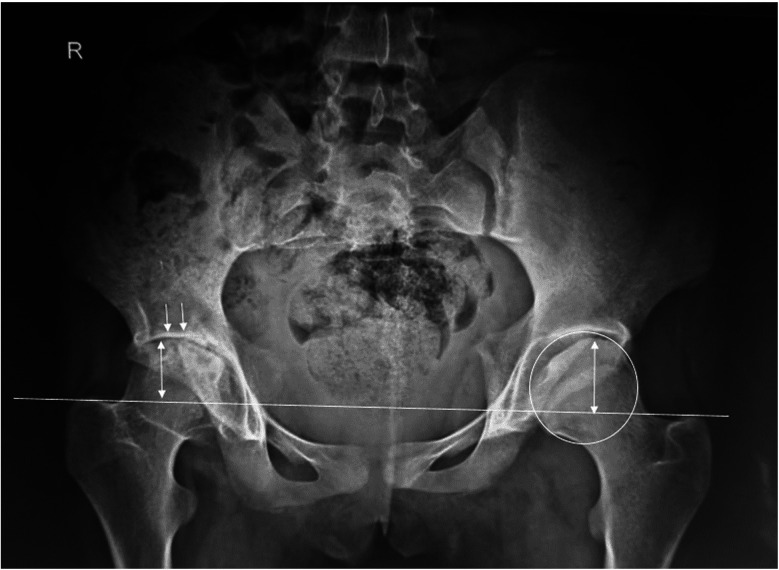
Bilateral hip radiograph of an 18-year-old girl with polyarticular JIA of 10 years duration. The radiograph showed bilateral joint space narrowing (white arrows), femoral head flatting (white circle), and asymmetric trochanteric femoral height.
